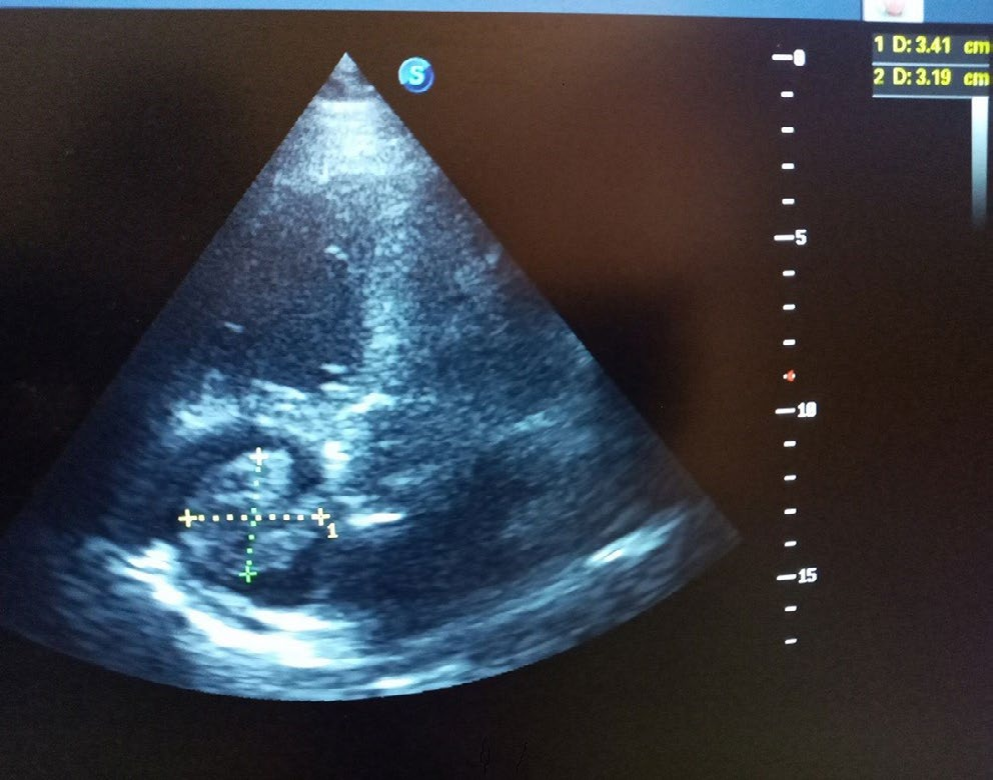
Two‐dimensional (2D) echocardiogram showing huge thrombus in the right atrium, measuring 34.1 × 31.9 mm
